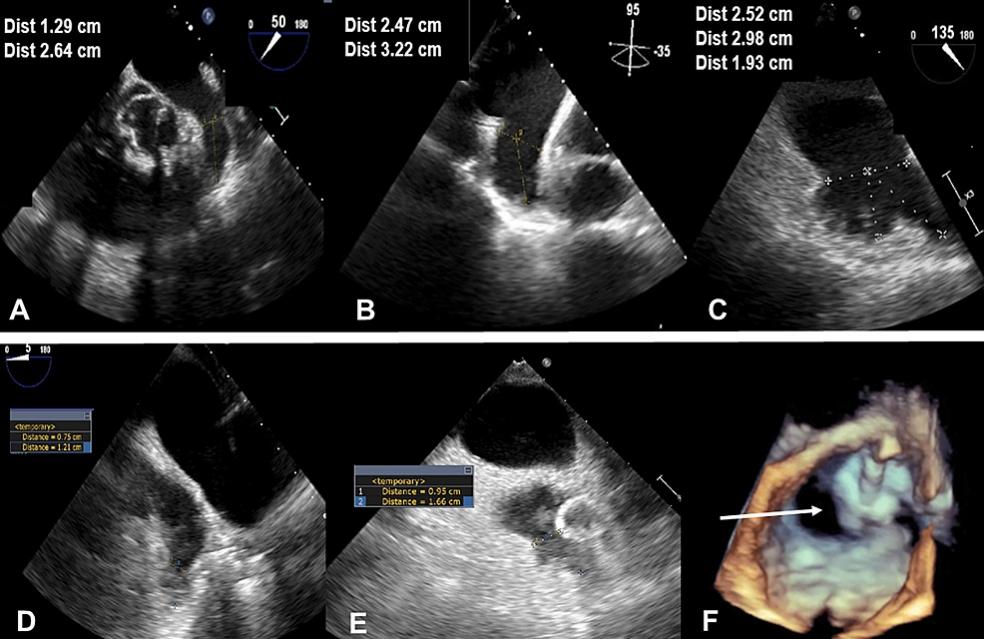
Multiplane transesophageal echocardiography during left atrial appendage closure Panels A-C: Multiplane TEE showing large bilobar LAA. Panel B: Demonstrates the longest depth measuring 3.22 cm. Panel C: Demonstrates the widest ostial diameter of 2.52 cm. Panels D-E: Show a moderate PDL size involving the posterior lobe measuring 9 mm in width by 16 mm in length. Panel F: 3D TEE showing moderate size PDL (arrow) in proximity to the Watchman occluder. TEE: transesophageal echocardiography; LAA: left atrial appendage; PDL: peri-device leak
